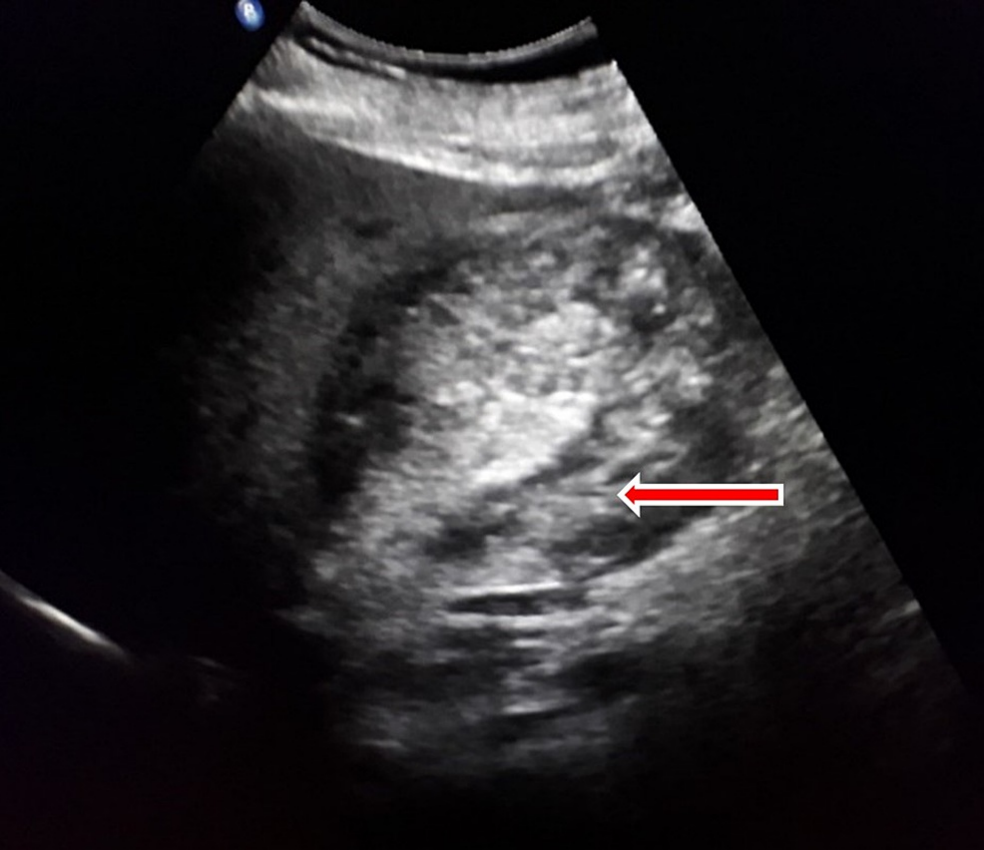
Transabodimal USG of the gallbladder. Mixed echogenic materials and non-acoustic shadowing USG, ultrasonography
